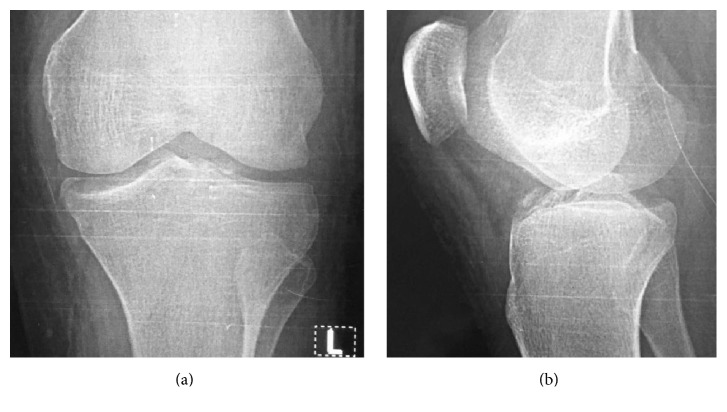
Anteroposterior (a) and lateral (b) postoperative radiographs. Medial and anteromedial rim fractures were repaired using nonabsorbable transosseous sutures. Tibial attachment of ACL was reduced and fixed in place using pullout sutures.
